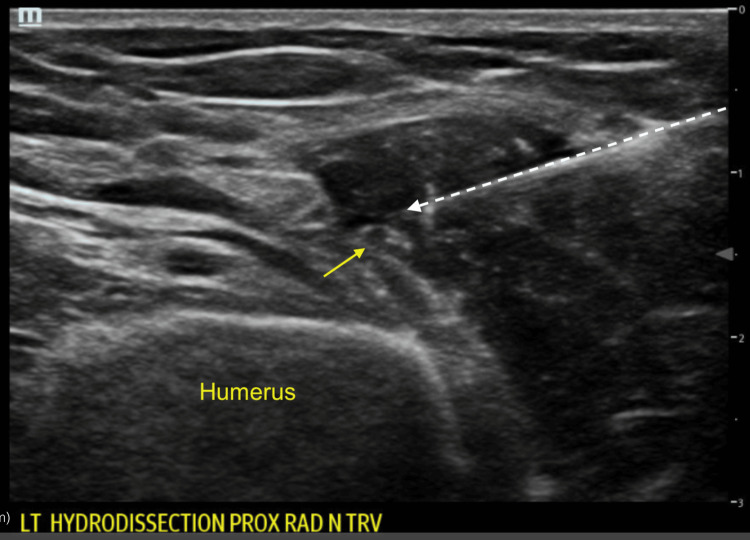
Transverse ultrasound view of early radial nerve hydrodissection showing the radial nerve (yellow arrow) and needle (white dotted arrow)
